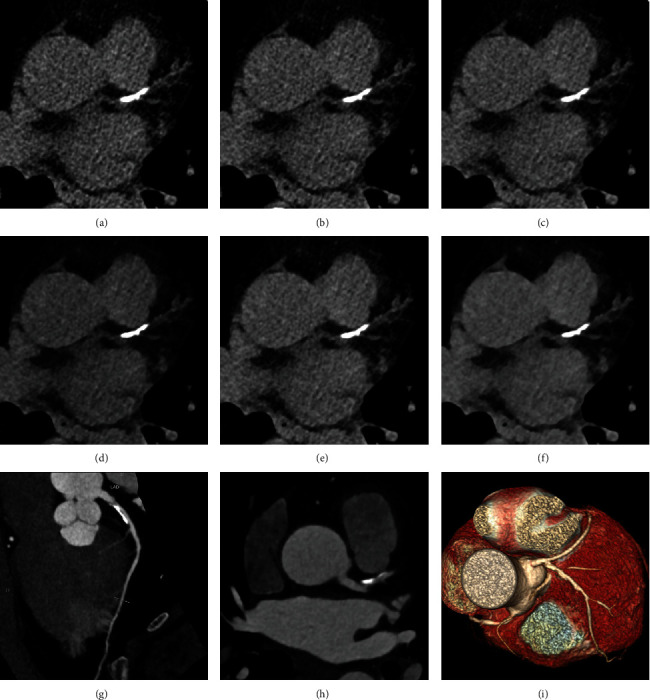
An example in a 71-year-old woman with suspected coronary artery disease. (a–f) Transverse CT images reconstructed with FBP (a), SAFIRE 1 (b), SAFIRE 2 (c), SAFIRE 3 (d), SAFIRE 4 (e), and SAFIRE 5 (f) and 3.0 mm reconstruction slice thickness. A calcified plaque located in the proximal segment of the left anterior descending artery is clearly shown in FBP and SAFIRE 1-5 images with the total Agatston score of 179.3, 163.9, 157.8, 154.2, 145.4, and 134.9, respectively. (g–i) Curved planar reformation of the left anterior descending artery (g), transverse CT image (h), and volume rendered reformatted image (i) in CCTA. A moderate stenosis caused by this calcified plaque is located in the proximal segment of the left anterior descending artery.
